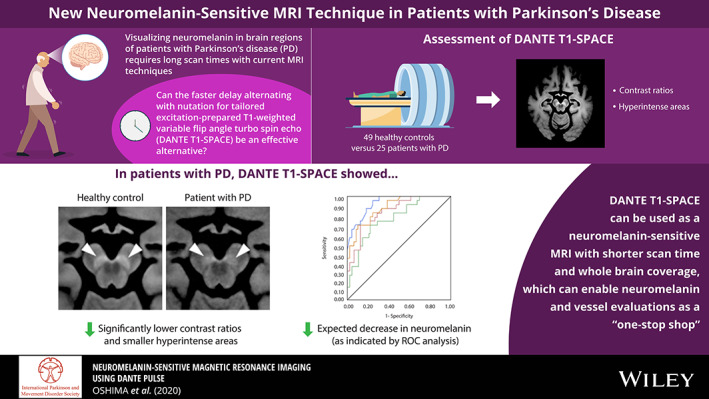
Parkinson's disease (PD) is a neurodegenerative disorder involving the progressive loss of dopaminergic neurons of the substantia nigra pars compacta (SNpc); these neurons contain a pigment called neuromelanin.^1^, ^2^ Neuromelanin is a strong chelator of heavy metals, particularly iron, and plays an important role in protection from toxicity caused by free iron.^3^, ^4^ The presymptomatic phase of PD often spans more than 20 years, and symptoms of PD are considered to appear when 50% to 60% of dopaminergic neurons degenerate.^5^, ^6^ Various two‐ and three‐dimensional neuromelanin‐sensitive magnetic resonance imaging (NM‐MRI) methods have been investigated to improve the early diagnosis of PD by exhibiting decreased signal intensity in the SNpc of patients with PD.^7^, ^8^, ^9^, ^10^, ^11^, ^12^, ^13^, ^14^, ^15^, ^16^, ^17^, ^18^, ^19^, ^20^ Magnetization transfer (MT) effects and T1‐shortening effects are considered to contribute strongly to the contrast of this imaging.^7^, ^8^, ^9^, ^10^, ^11^, ^12^, ^13^, ^14^, ^15^, ^17^, ^18^, ^19^, ^21^ Previous studies have shown more prominent reduction in signal intensity in the SNpc as PD advances.^7^, ^17^ Another study found that NM‐MRI reflects the function of SN dopamine neurons.^13^ However, these imaging techniques require relatively long scan times (8–12 minutes) despite limited slice coverage, causing difficulty in obtaining sufficient image quality from patients with tremor or involuntary movements.^7‐10,12‐15,17‐19^
